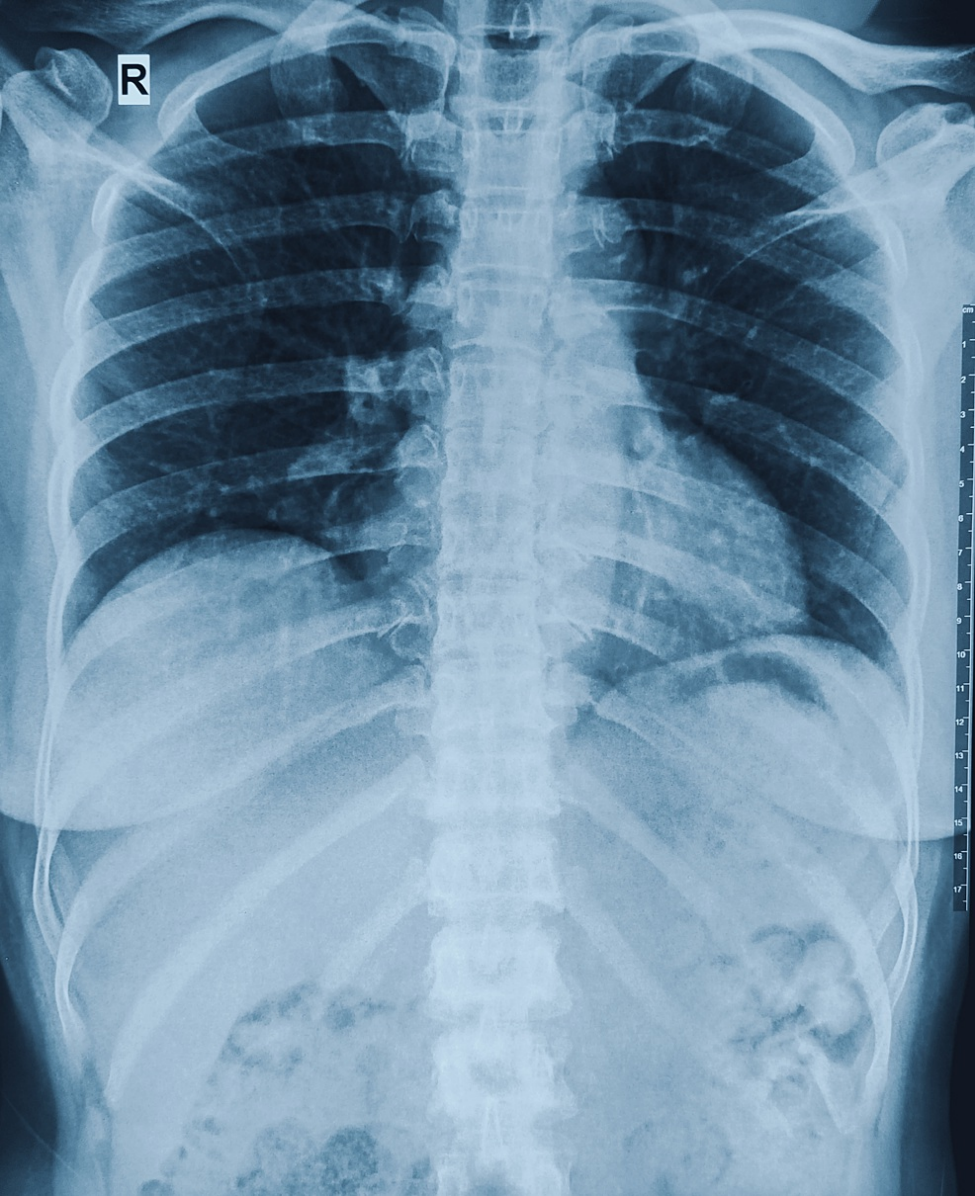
Chest X-ray showed no abnormalities.
